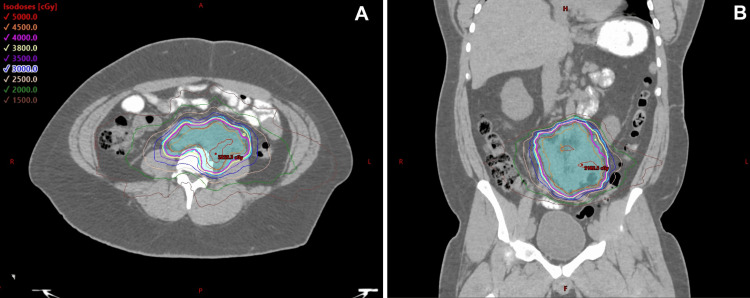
Isodose distributions in the axial (A) and coronal (B) planes of the CT planning dataset with a prescription of 40 Gy in five fractions. Anterior and lateral borders of the PTV (cyan) are undercovered to spare the small bowel and ureter. CT, computed tomography; PTV, planning target volume
